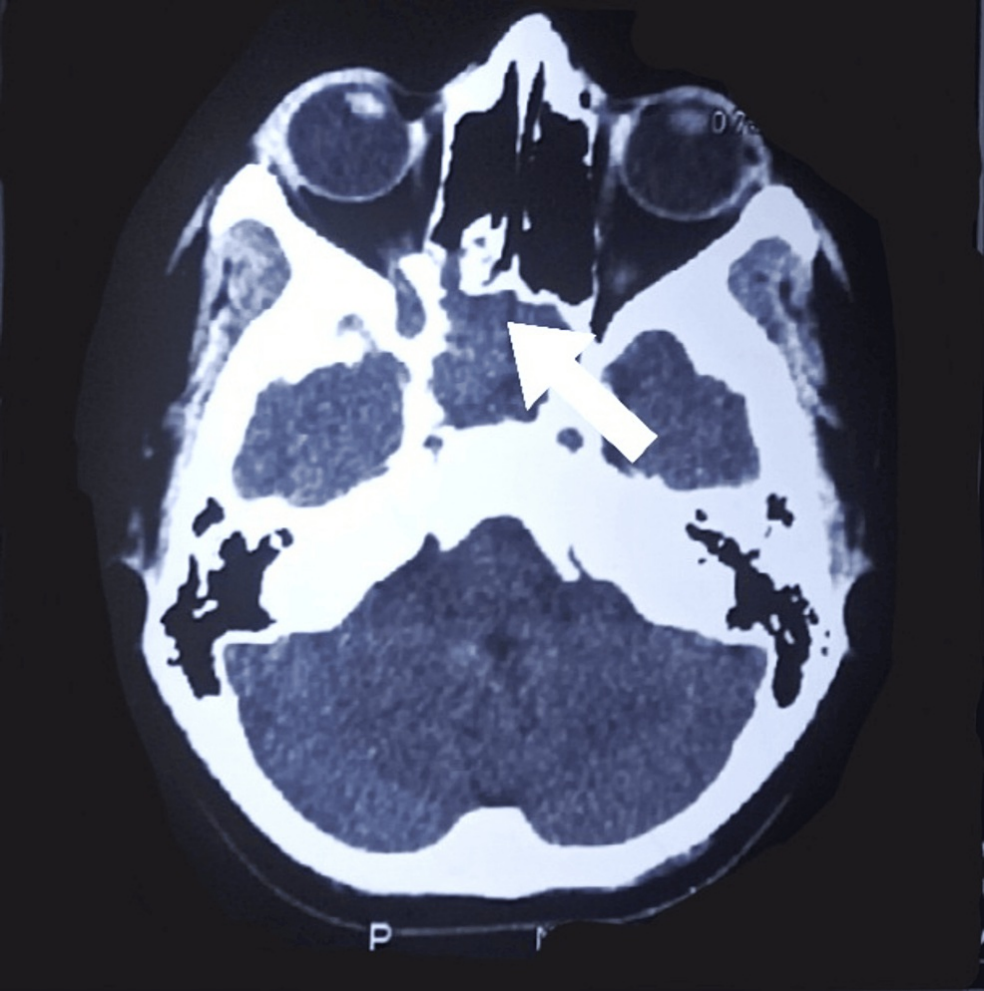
CT scan of the nose and paranasal sinuses showing the lesion extending into the right posterior nasal cavity as pointed by the arrow
